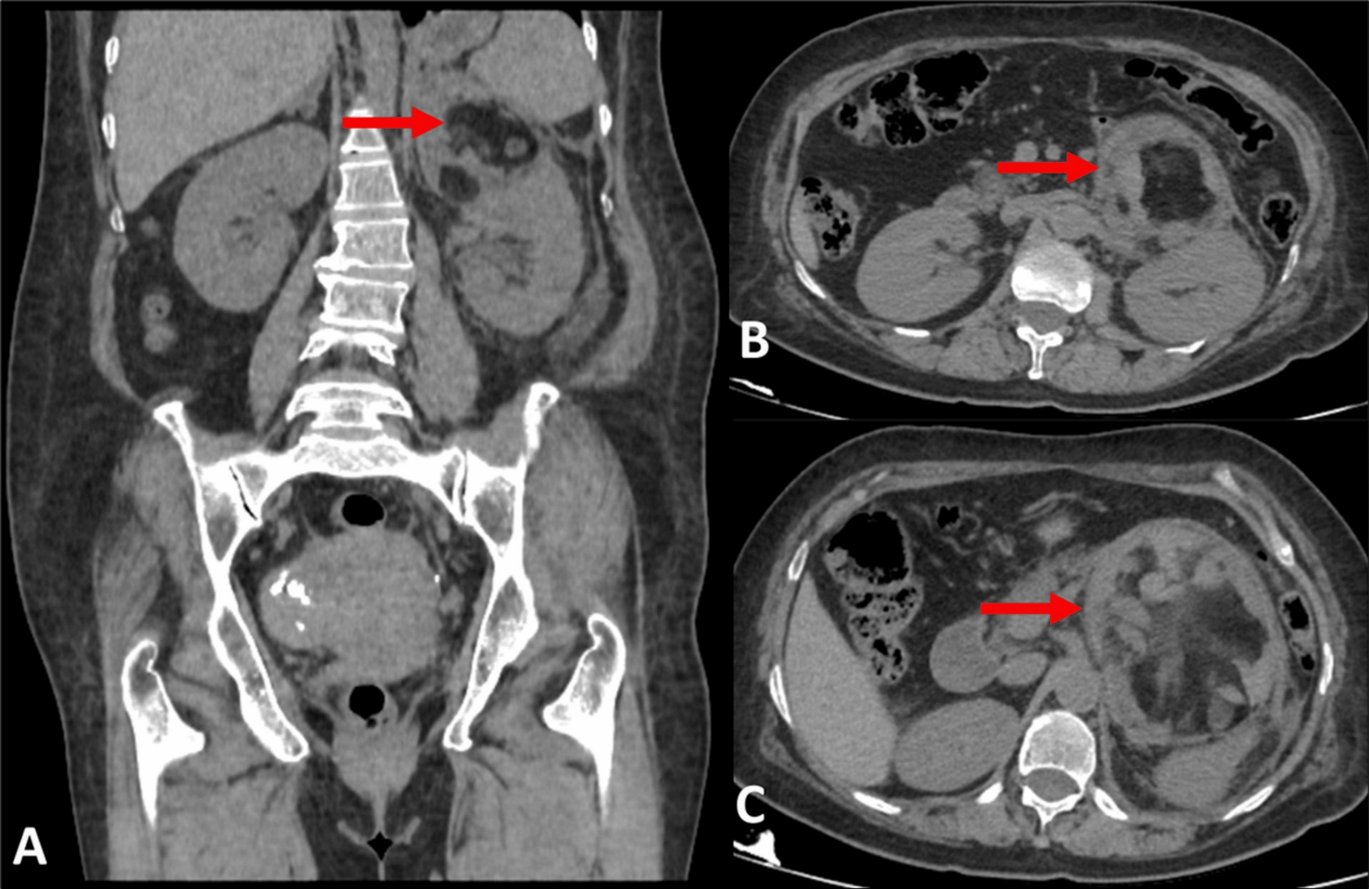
Non-contrast-enhanced computed tomography images. (A) Coronal section showing a retroperitoneal mass in the anterosuperior aspect of the left kidney. (B) Axial section showing the same retroperitoneal mass. (C) Axial section showing the mass abutting the tail of the pancreas.
